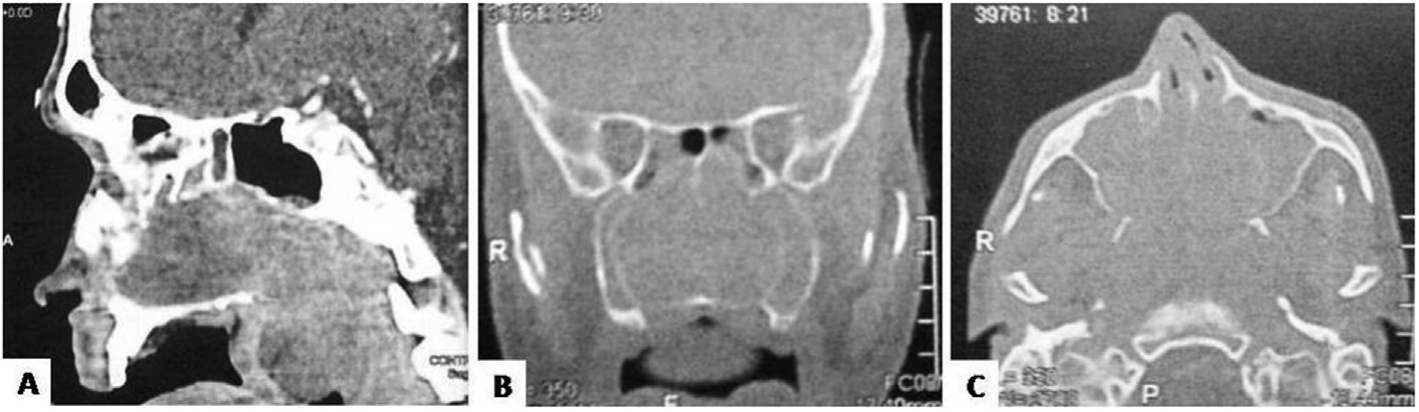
Computed tomography showing sagital (A), coronal (B) and axial (C) views, respectively.
